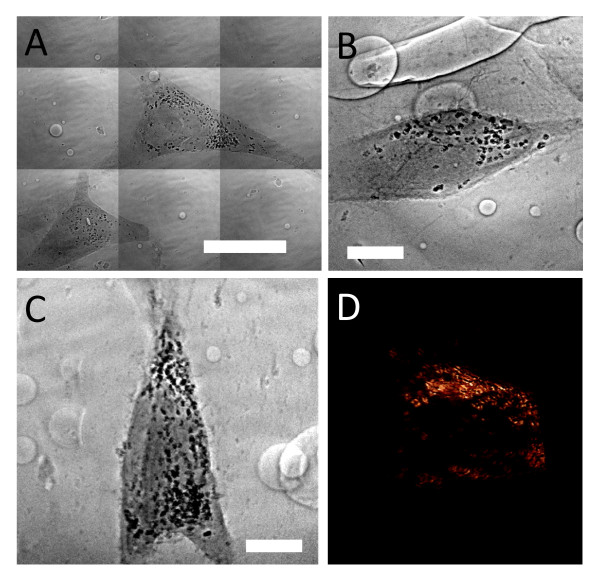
Transmission x-ray microimages of 3D cultured HeLa cells. Similar to Figure 7, the HeLa cells were grown on an OPLA scaffold. Bar in A: 20 μm, B and C: 5 μm. (Additional files 4 (9A) and 5 (9B))
